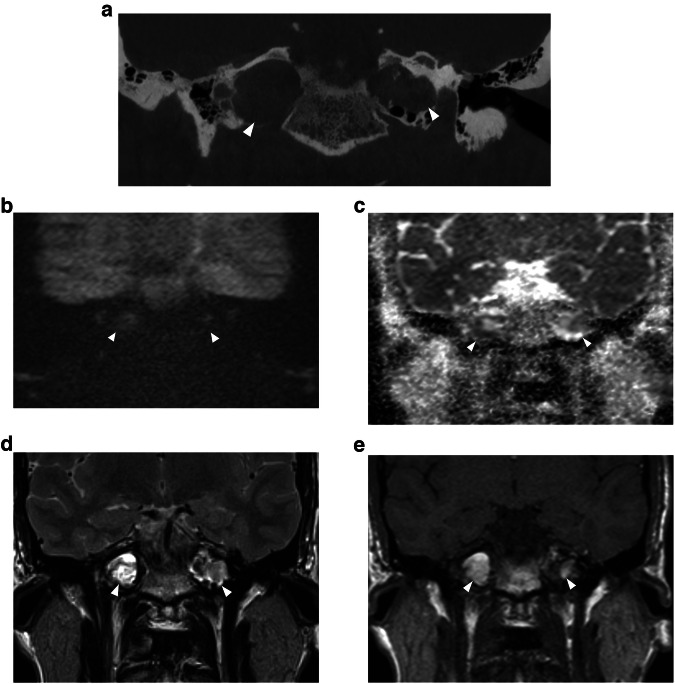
Bilateral cholesterol granuloma of the petrous apex in a 34-year-old man. a Coronal reformatted CBCT image showing a sharply delineated lytic lesion in the petrous apex on both sides (white arrowheads). b Coronal b1000 DWI, (c) ADC map, (d) T2-WI and (e) T1-WI. The lesions in both the petrous apices (white arrowheads) have a heterogenous signal on DWI and ADC with some signal hyperintensity owing to T2 shine-through. The lesions have an intermediate to high signal intensity on T2-WI. The high signal intensity on T1-WI, however, is almost pathognomonic for a cholesterol granuloma
